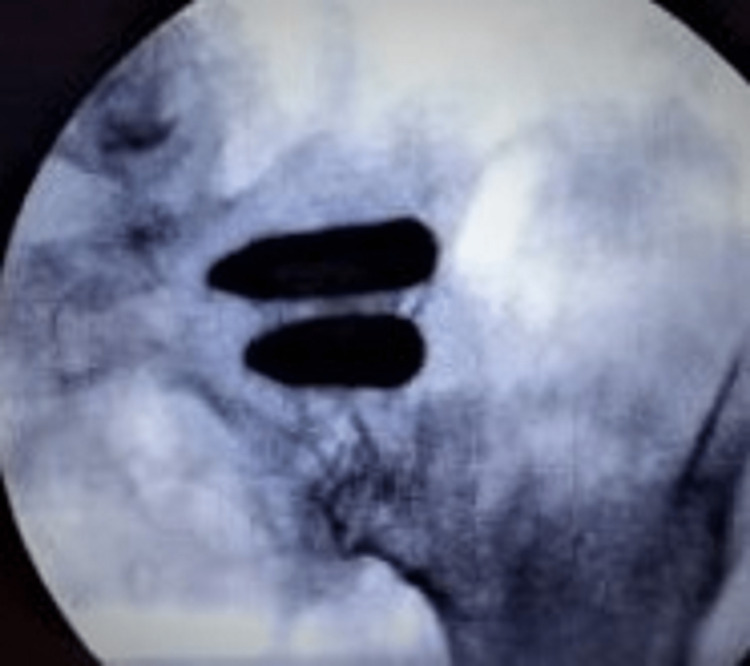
Radiographic image of the same patient (patient 2, as shown in Figure 4) 8 months after fusion. The implants look solid, without any empty spaces within them, and the screw threads are completely blunted indicating bony fusion.
